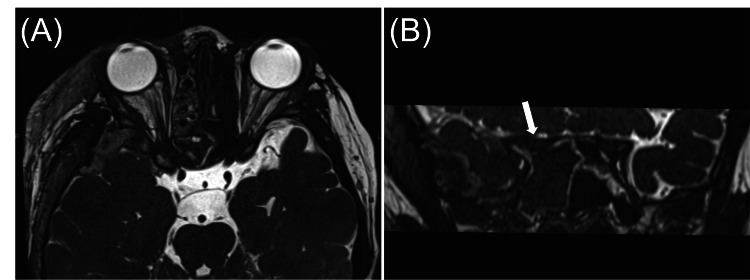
Constructive interference in steady state (CISS) magnetic resonance imaging (MRI) of the orbit on postoperative day 2 MRI (A: axial view; B: coronal view) showing no abnormalities in the right intra-orbital and optic nerve (white arrow).
